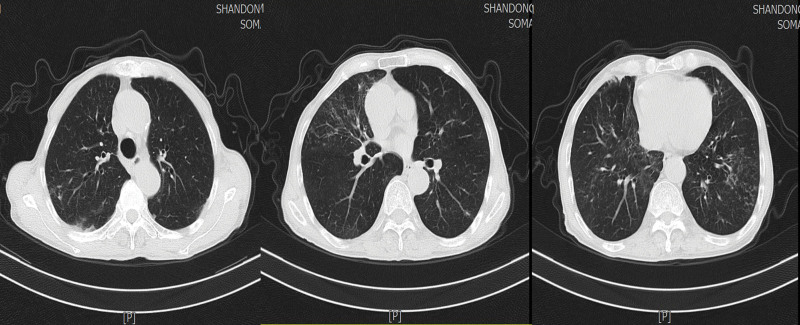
Chest CT performed on March 30, 2023 showing that the lung lesions had improved markedly. CT = computed tomography.
